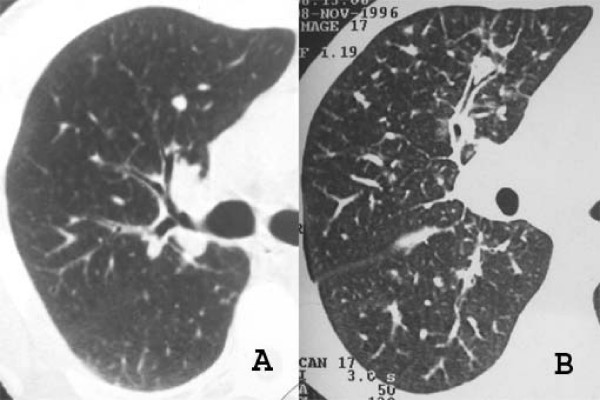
A and B. High-resolution CT scans of two patients with pulmonary KS showing peribronchovascular thickening and irregular narrowing of the bronchial lumen.
